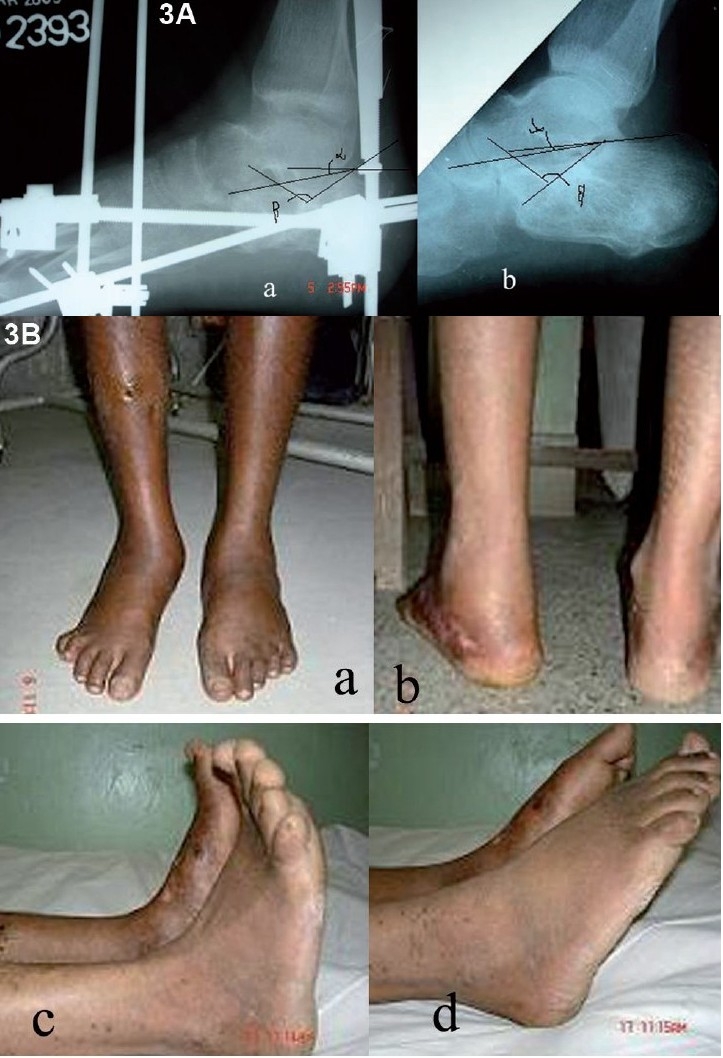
(A-B): (A) (a) X-ray of the ankle(lateral view) just before fixator removal showing evidence of bone healing with correction of altered calcaneal angles achieved by ligamentotaxis. (b) X-ray of the ankle of the same patient (lateral view) at one-year follow-up showing subtalar joint without any collapse of calcaneal angle correction, achieved by ligamentotaxis (B) Clinical Photograph of the same patient at final followup showing (a) full weight-bearing, (b) no significant clinical heel deformity, (c) and (d) good range of ankle movements achieved
